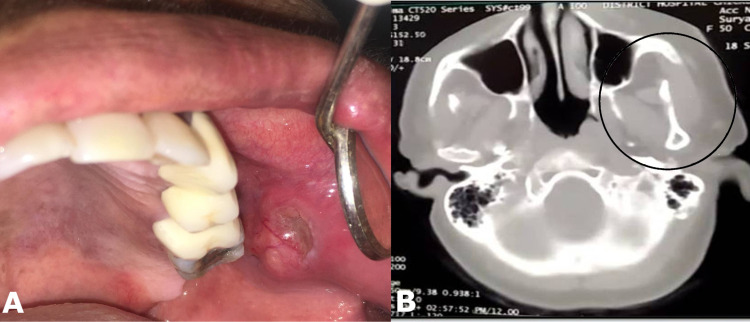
(A) Clinical image showing a nodular cystic swelling of around 2 cm opposite to left maxillary second premolar and first molar teeth region on the buccal mucosa. (B) Computed tomogram (CT) scan showing normal maxillary bone and maxillary antral areas with no bony involvement by the tumour (area highlighted by a circle).
